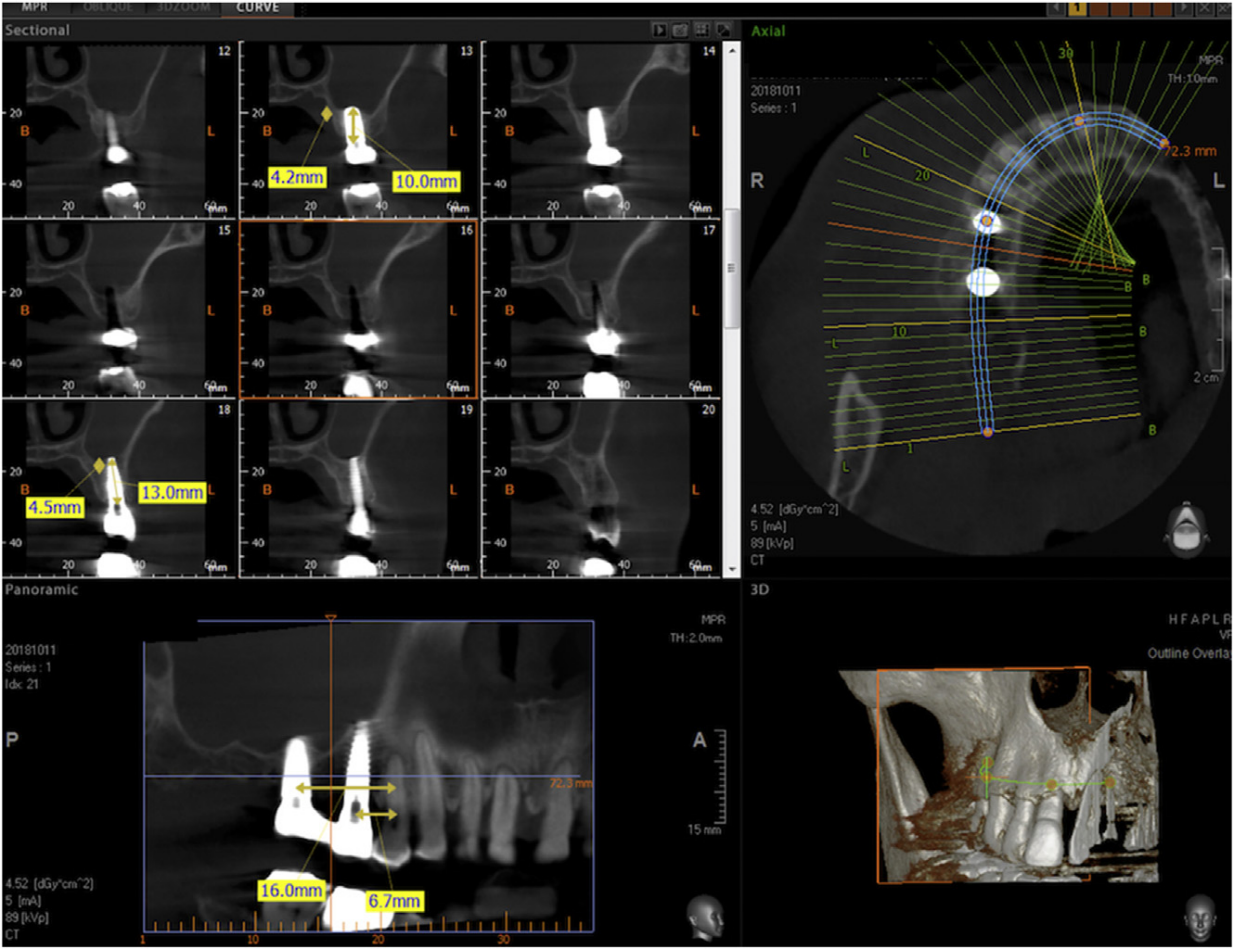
Sectional CBCT of the Flapless LMSF case shown in figure 11 taken one year after surgery and 10 months after prosthetic loading. Both sites show evident vertical bone gain below the Schneiderian membrane. The implant in the first molar position is 4.8 × 10 mm and the implant in the second bicuspid position is 3.8 × 13 mm. Both are Sweden&Martina Global UXR.
